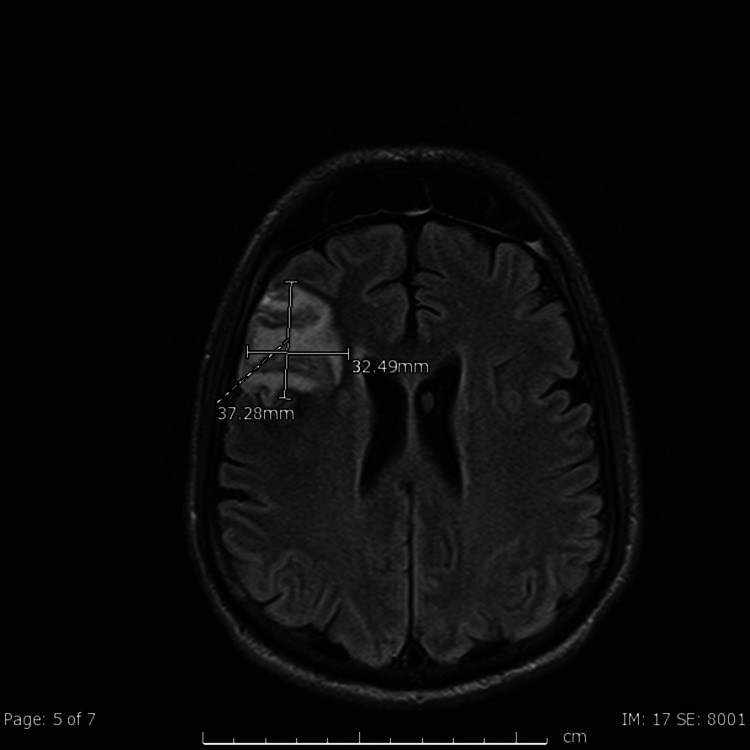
Axial FLAIR MRI of head demonstrating multifocal areas of acute infarct involving the right temporal lobe and right frontoparietal junction FLAIR, fluid-attenuated inversion recovery
